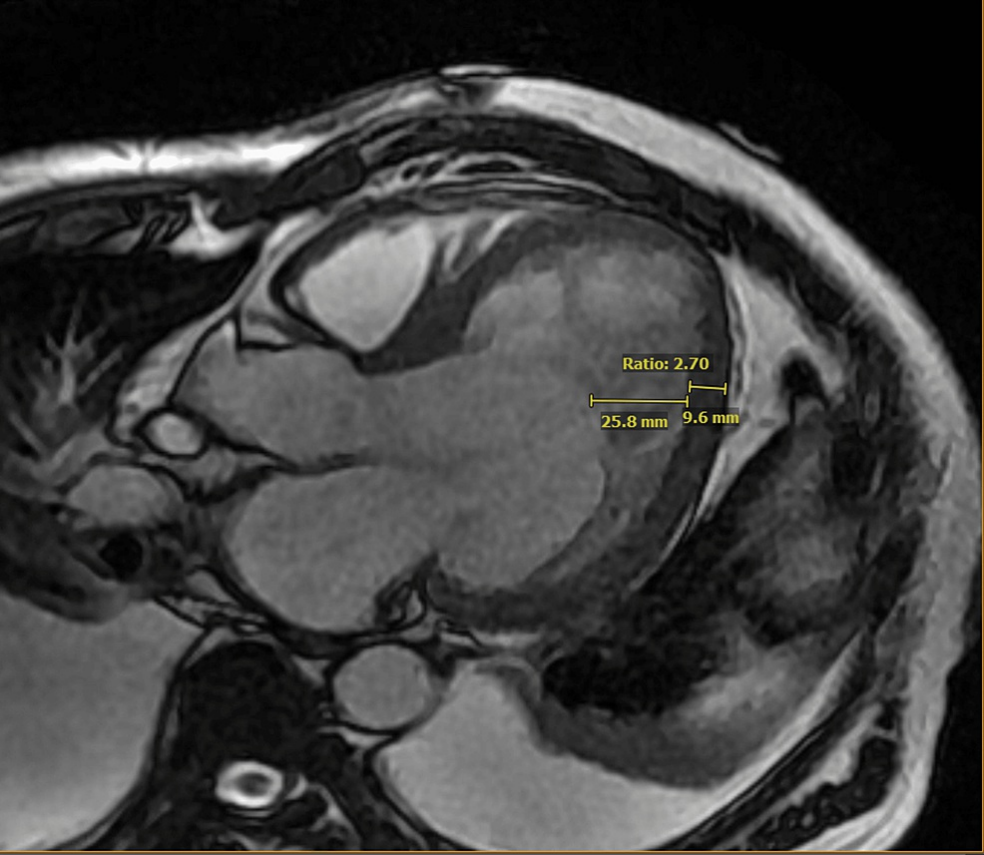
Cardiac MRI showing non-compaction cardiomyopathy This is a long-axis view of cardiac MRI in the end-diastole phase. It shows a non-compaction to compaction ratio of greater than 2.3 thus diagnosing NCCM (Peterson's criteria) NCCM: Non-compaction cardiomyopathy
